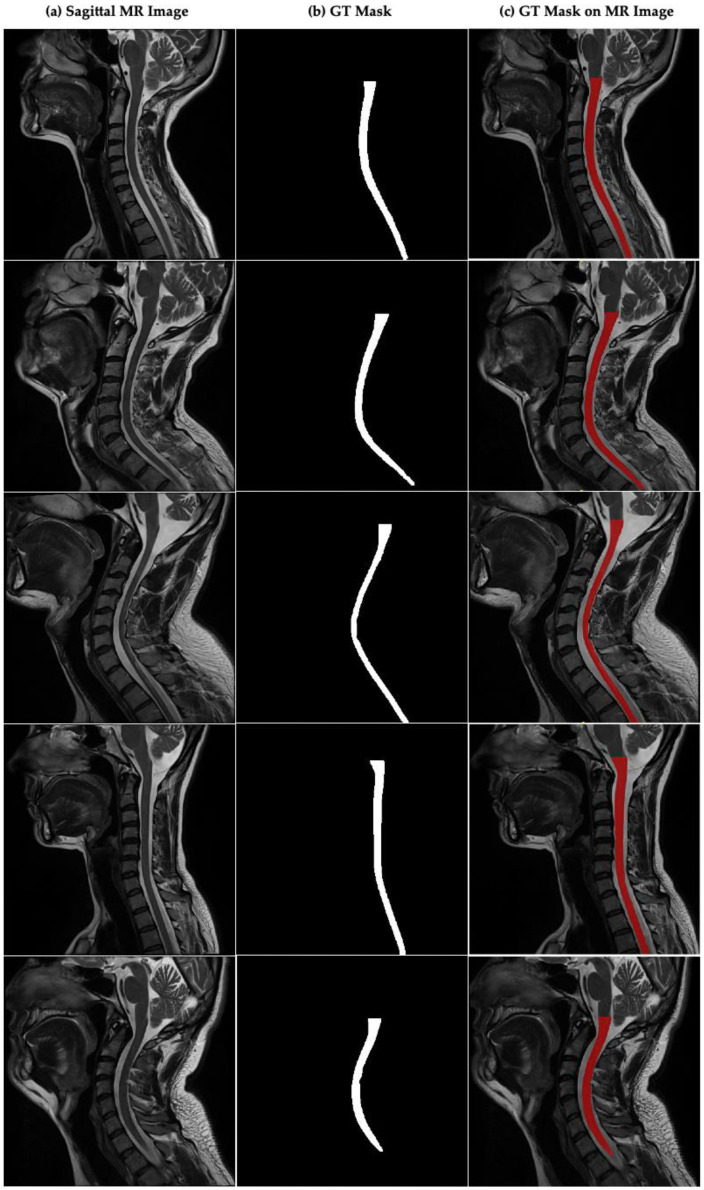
Sagittal plane MR image of the cervical spinal cord and CSA boundaries annotated by the experts in cervical spinal cord. (a) Sagittal MR images in dataset, (b) GT mask annotated by experts for cervical spinal cord, (c) GT mask of CSA on sagittal MR image.
